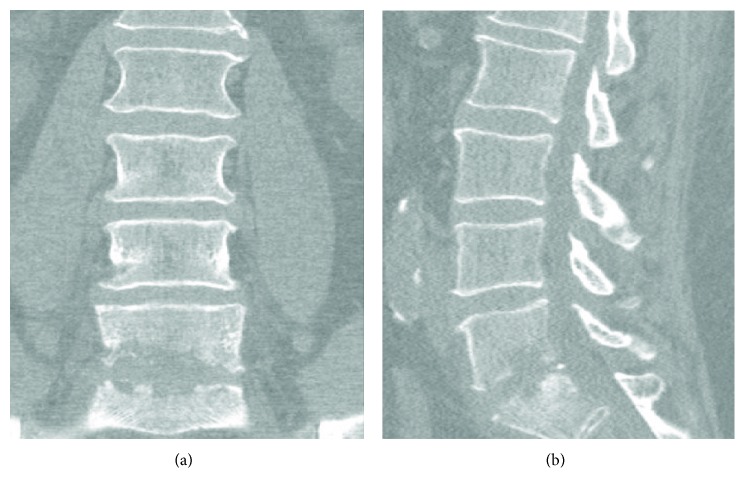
CT imaging of lumbar spine approximately 5 weeks after initial fall. Evidence of significant discitis and end plate destruction at L5/S1 level.
